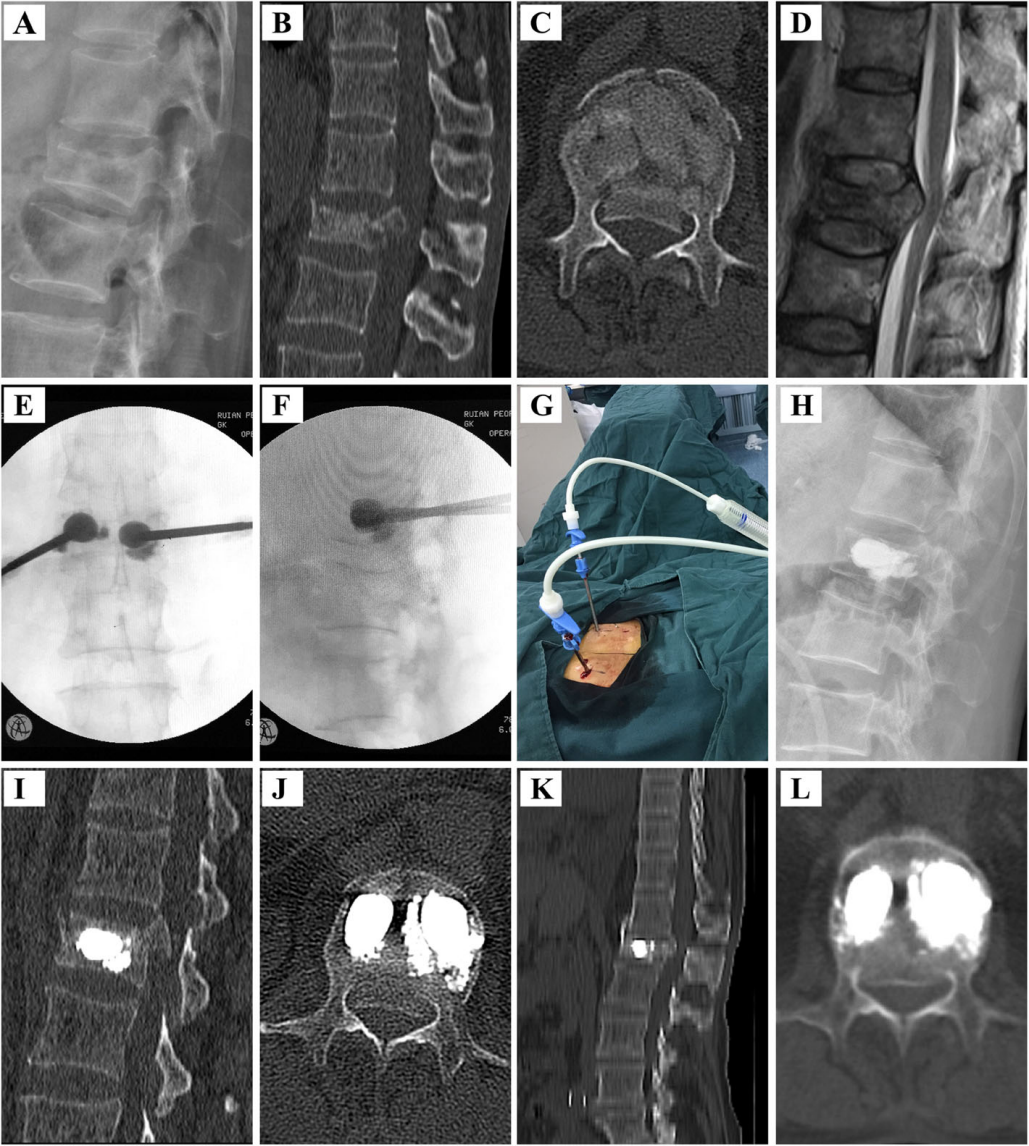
PMCP surgical procedure for the treatment of a 65-year-old female patient with OVBFs in L_1_ vertebra. A Preoperative lateral radiograph showing a burst fracture of L_1_. B, C Preoperative CT-scan (plain and sagittal reconstruction image) showing the burst fracture with spinal canal compromise. D Preoperative MRI (T2-weighted sequences) showing the burst fracture with spinal canal compromise. E, F Intraoperative fluoroscopic image demonstrating PMCP surgical procedure. G Intraoperative view. H Postoperative lateral radiograph showing better alignment following cement injection and adequate vertebral body reduction. I, J Postoperative CT-scan (plain and sagittal reconstruction image) showing no worse spinal canal compromise and better alignment and adequate vertebral body reduction. K, L CT-scan (plain and sagittal reconstruction image) 2 years after surgery showing reduced spinal canal compromise, excellent fracture healing and alignment
